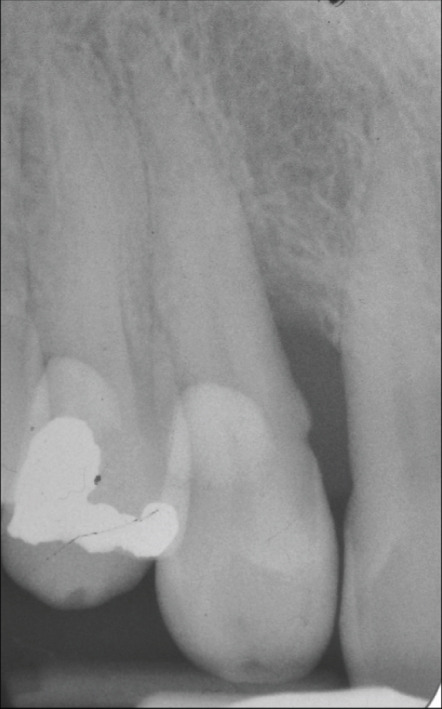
Radiograph at the level of the site with an infraosseous defect at baseline.
